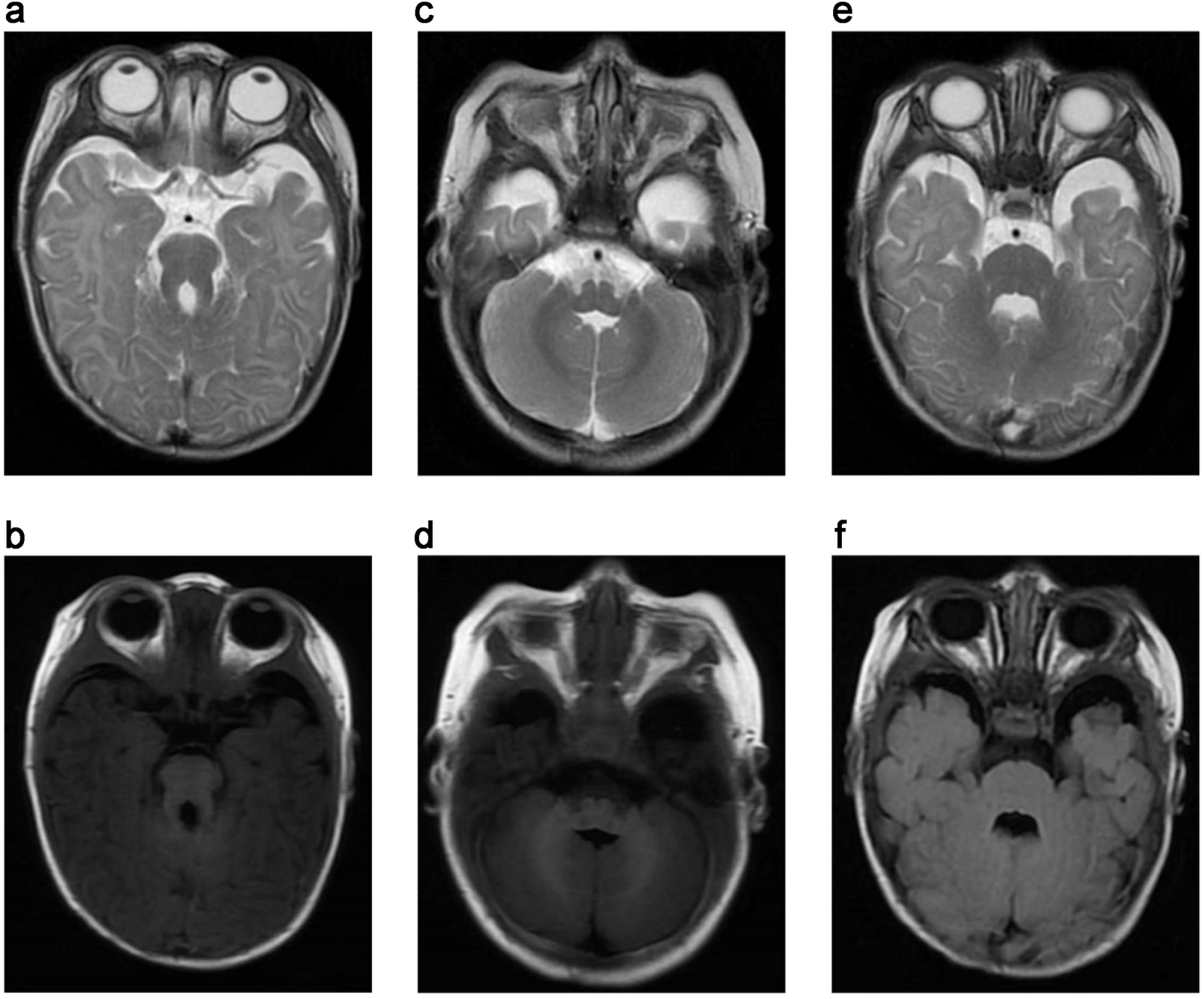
Brain MRI of the affected patient showing evidence of a molar tooth sign (a, T1WI; b, T2WI), dysplastic cerebellar vermis (c, T1WI; d, T2WI), and the typical “bat wing” morphology (e, T2WI; f, T2 fluid-attenuated inversion recovery sequence axial MRI).
